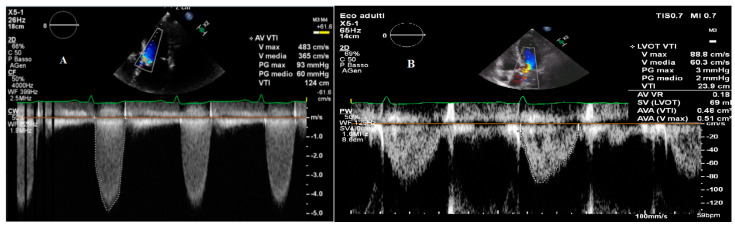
(A) Recording of the peak velocity through a stenotic aortic valve in the apical five-chamber view by continuous-wave Doppler. (B) Recording of the peak velocity through Left ventricular outflow tract in the apical three-chamber view by pulse-wave Doppler. The images were executed with Philips EPIQ 7 (Philips Medical System, Bothell, WA, USA).
